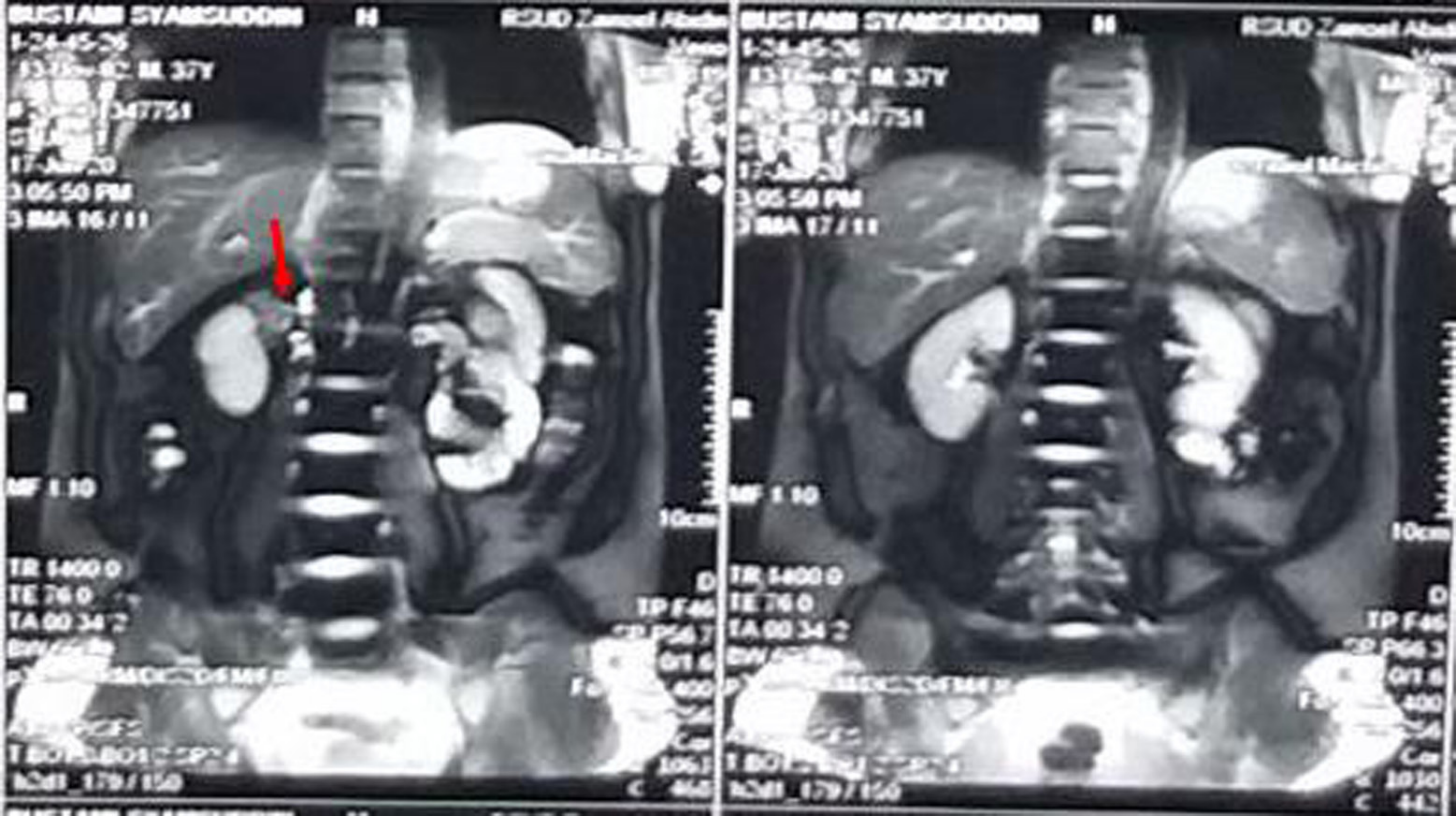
Abdominal MRI examination showed right-side adrenal enlargement and calcification (red arrow).
